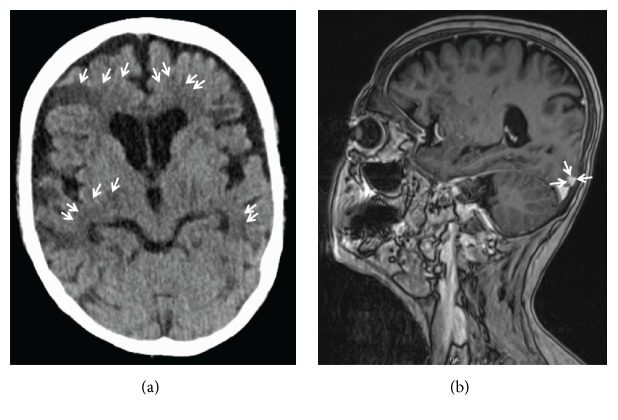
Bilateral ischemic lesions (CT, (a) arrows) and contrast-enhancing lesions directly adjacent to the dura mater (MRI, (b) arrows), radiologically consistent with multiple septic emboli and tuberculosis manifestation within the CNS.
